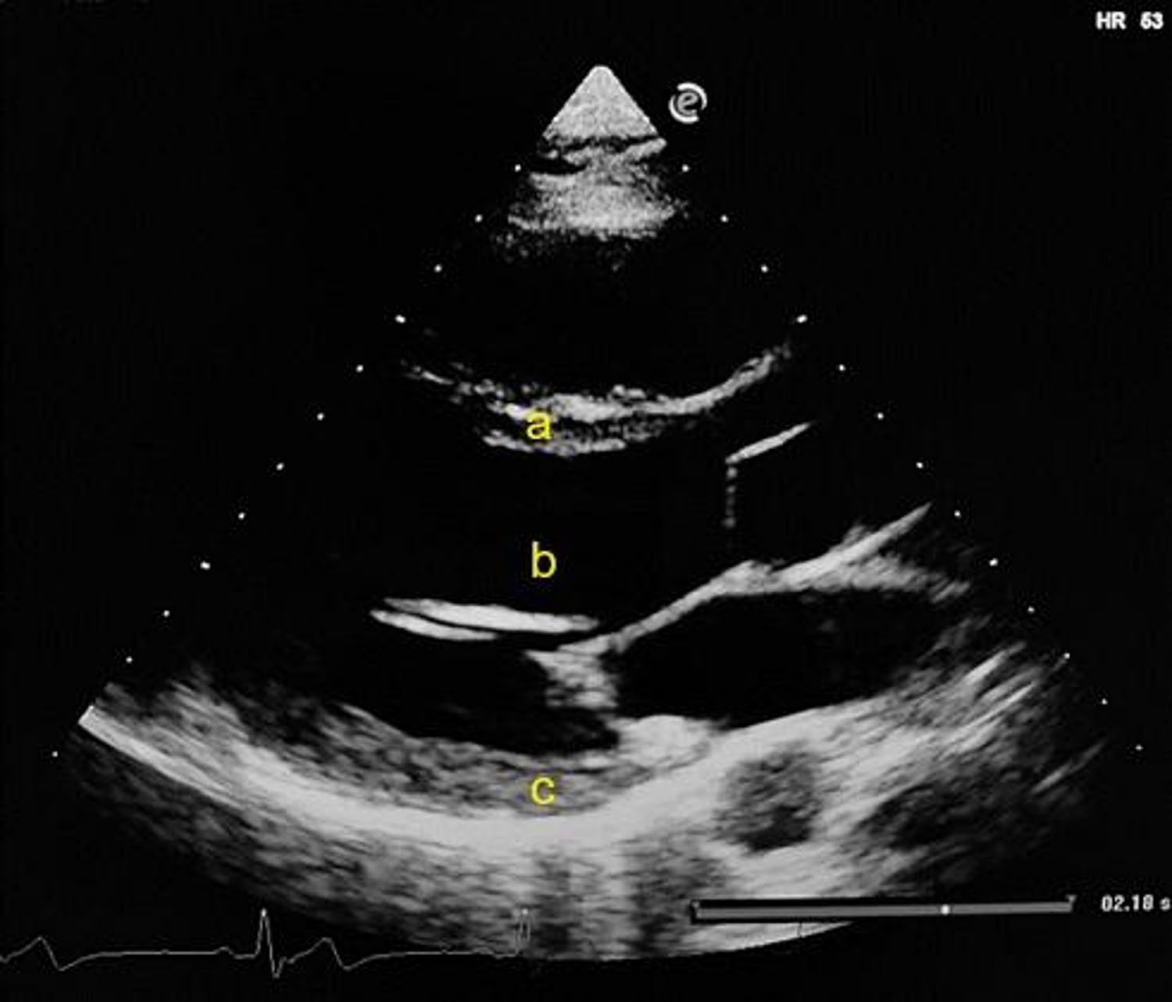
Sample echocardiographic image of a parasternal long-axis view acquired in the present study. Labels indicate key measurements: a interventricular septum (IVS), b left ventricular internal diameter (LVID), and c posterior wall (PW). All measurements were taken at end-diastole, defined the frame before mitral valve closure or the frame in the cardiac cycle in which the ventricular dimension or volume is largest, as recommended by Lang et al. (2015)
